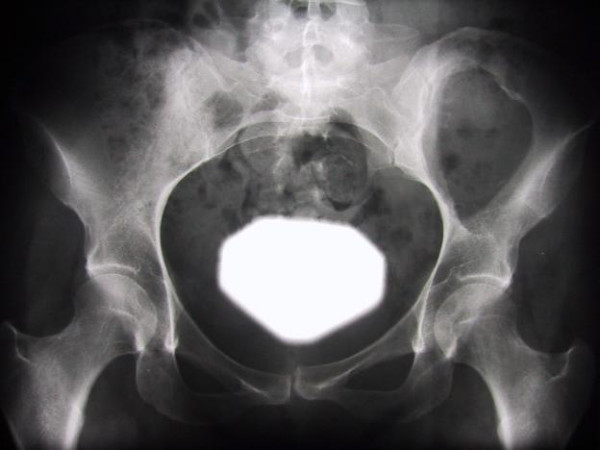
Radiograph of the pelvis showing a well-circumscribed osteolytic lesion in the left iliac bone; it is sharply marginated with a thin sclerotic rim and without any matrix calcifications.
